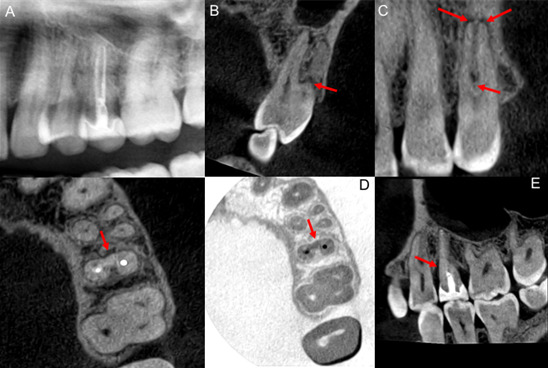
Caso 3. Detalle de radiografía panorámica donde no se evidencian signos radiológicos de fisura o lesión periapical. Corte coronal (B) y corte sagital (C) de tomografía computarizada de haz cónico en donde se evidencia imagen hipodensa en región de furca y región periapical del diente 2.4, y una línea hipodensa compatible con fractura. Cortes axiales (D) y sagital (E) que muestran el defecto óseo mesial en la unidad dentaria 2.5.
